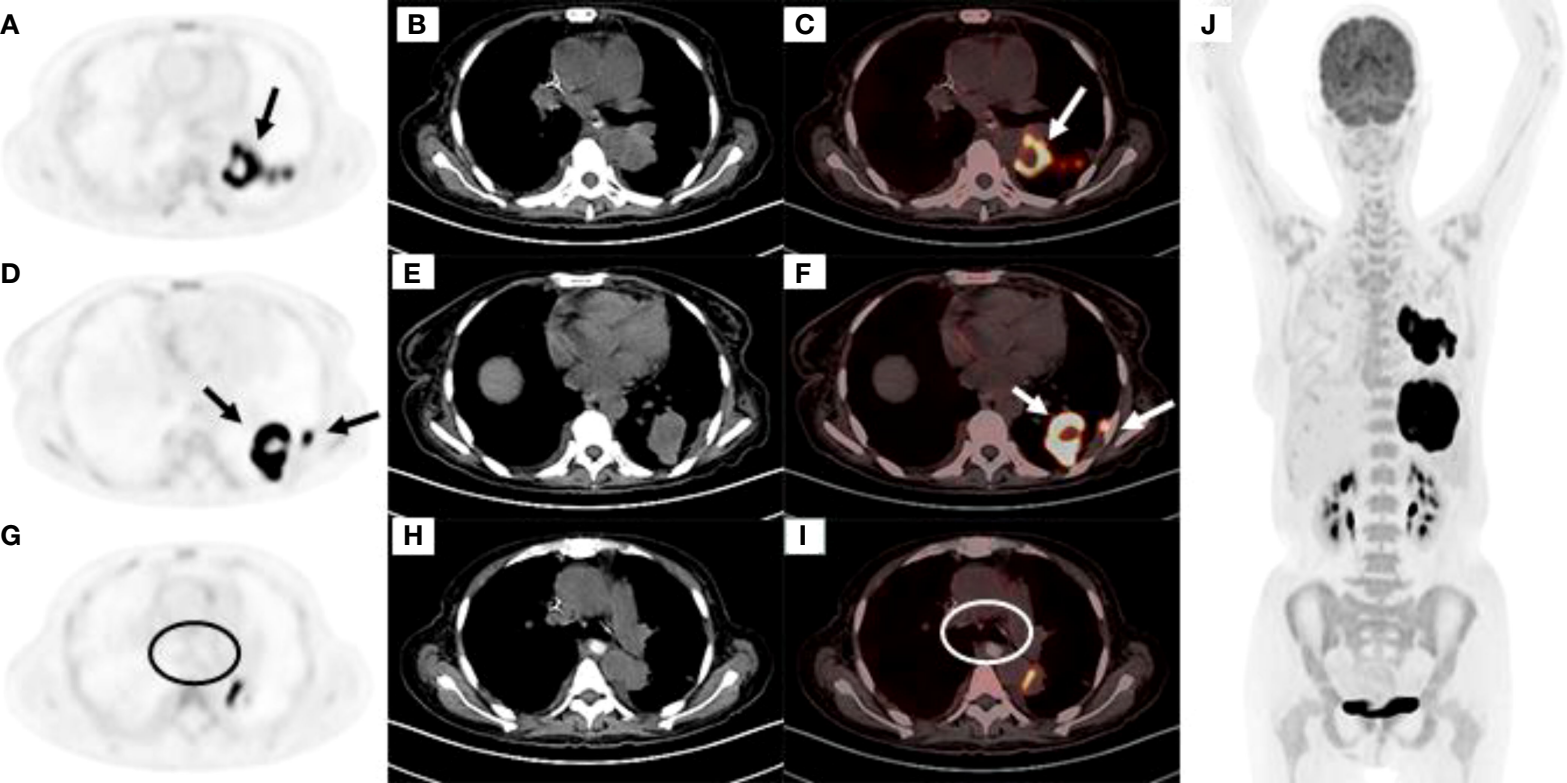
(A‒C) High FDG uptake at the dorsal segment of the left lower lobe (A) PET image, (B) CT mediastinal window, (C) PET and CT fusion images. (D‒F) High FDG uptake at the posterior basal segment and lateral basal segments of the left lower lobe (D) PET image, (E) CT mediastinal window, (F) PET and CT fusion images. (G‒I) No FDG uptake was observed in the mediastinal lymph nodes as shown within the circle (G) PET image, (H) CT mediastinal window, (I) PET and CT fusion images. (J) Except for the left lung tumor, there was no obviously high metabolism in other parts of the body.
